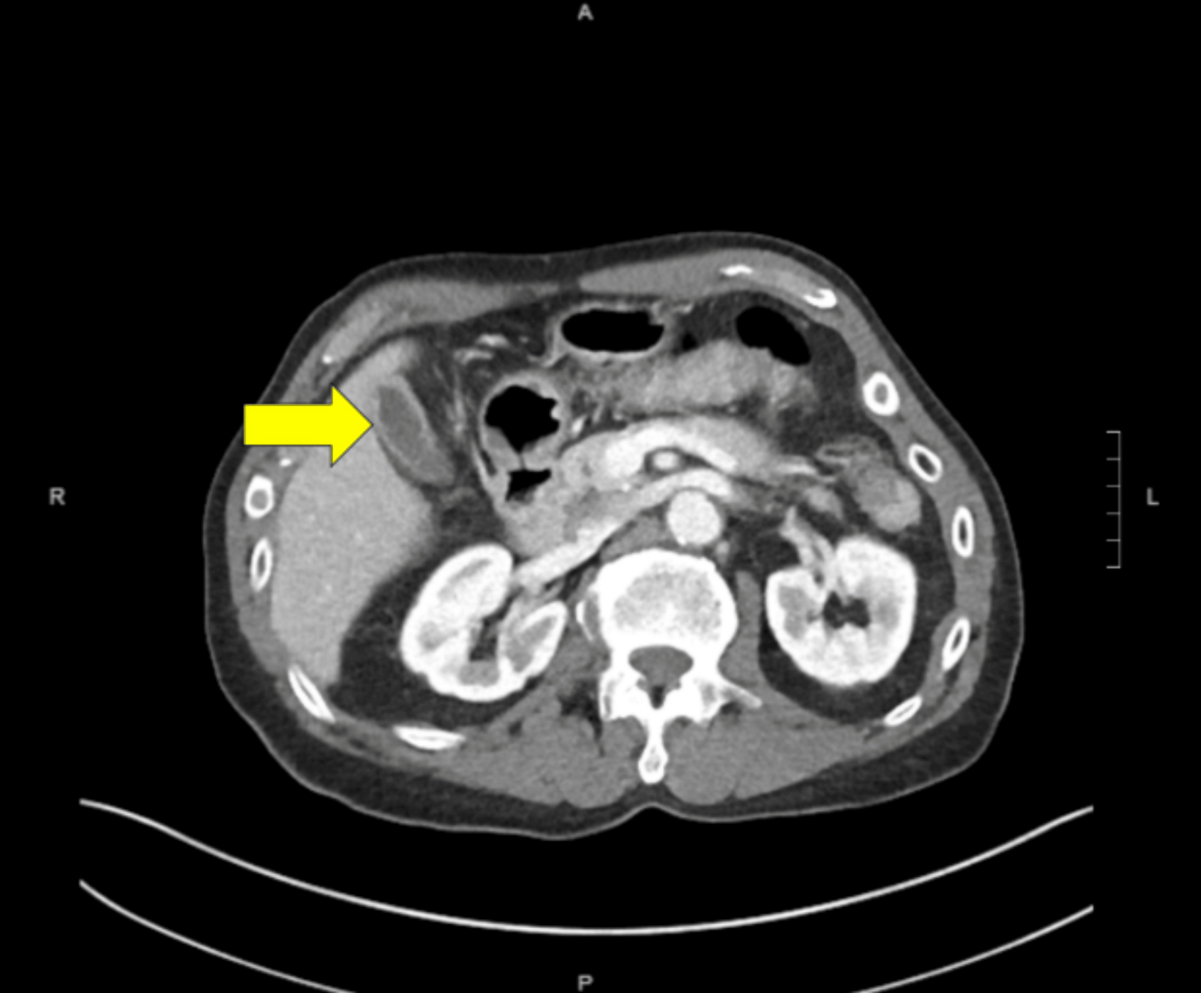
Transverse section of CT scan showing gallbladder with increased wall thickening (yellow arrow)
